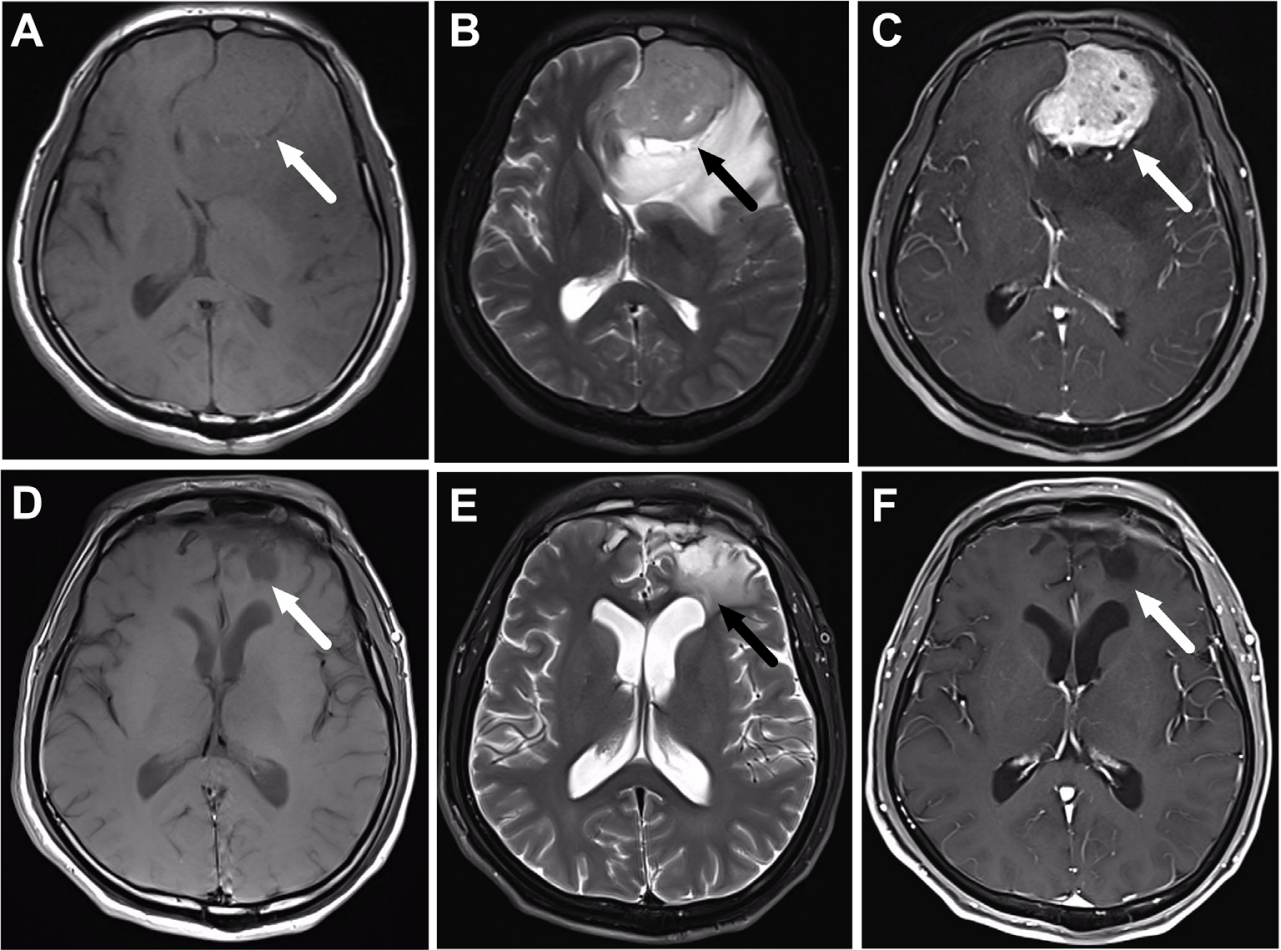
A 56-year-old male patient with olfactory neuroblastoma of Kadish Stage C underwent surgical resection followed by chemoradiotherapy. (A–C) are the magnetic resonance (MR) images before treatment, while (D–F) are the corresponding MR images after the completion of treatment. (A) Axial T1-weighted MR imaging reveals an isointense mass in the frontal lobe (arrow). The mass has moderate signal intensity on T2-weighted MR images (B), and shows heterogeneous enhancement on the post-contrast T1-weighted MR images (C). (D–F) show that the tumor totally disappears after the completion of treatment, and there is still edema of the frontal lobe (arrow).
